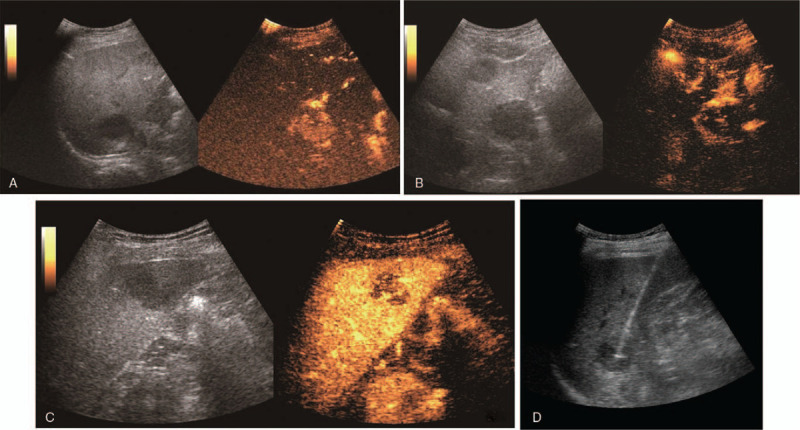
Contrast-enhanced ultrasonography and ultrasound-guided puncture of the tubercle. (A) Contrast-enhanced ultrasonography of nodule 1. (B) Contrast-enhanced ultrasonography of nodule 2. (C) Contrast-enhanced ultrasonography of nodule 3. (D) Ultrasound-guided puncture image.
